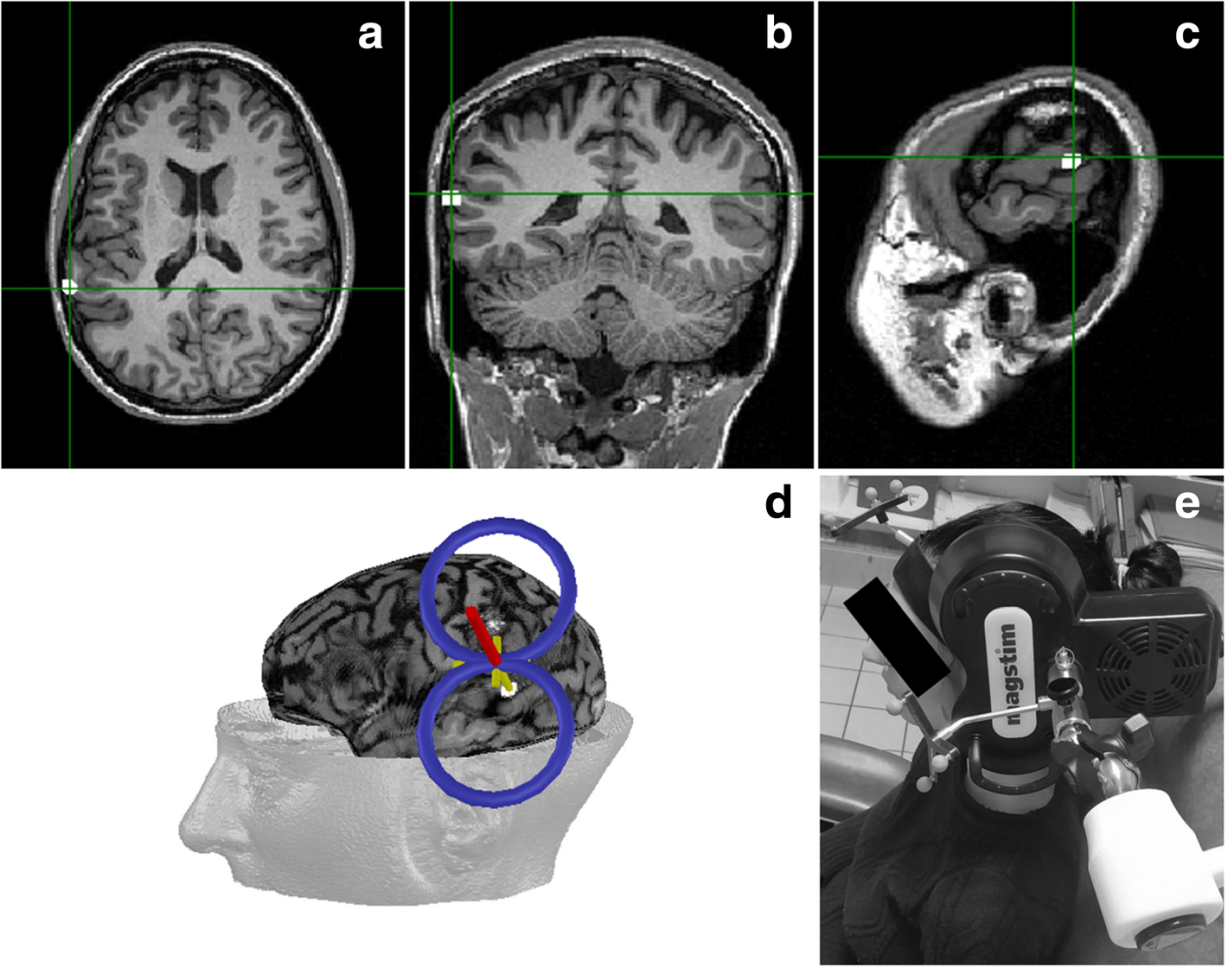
TMS-targeted region and coil placement. The left TPJ will be targeted using established spatial coordinates for the Wernicke’s area in the left TPJ (Montreal Neurological Institute (MNI) coordinates: x = − 69, y = − 41, z = 11; Hoffman et al. [25]). Axial (a), coronal (b), and sagittal (c) MRI views of the location for the TMS targeted left TPJ are shown in this figure. This brain area will be labeled on a 3D rendering of the patient’s individual T1 MRI sequence on each patient. The TMS coil will be positioned tangential to the scalp location overlying the left TPJ using an MRI-based frameless stereotactic neuronavigation system (d and e)
